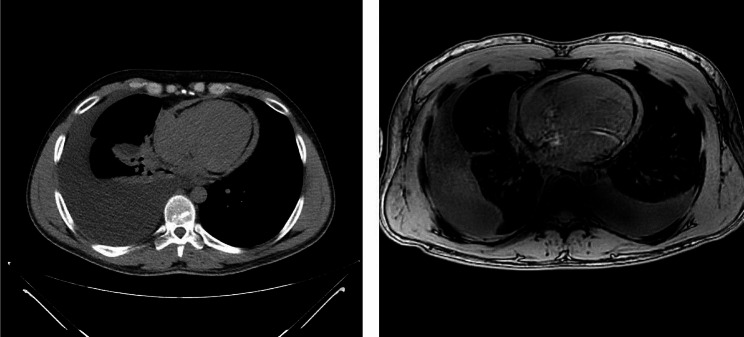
A and B CT scan and MRI demonstrating pericardial thickening.
